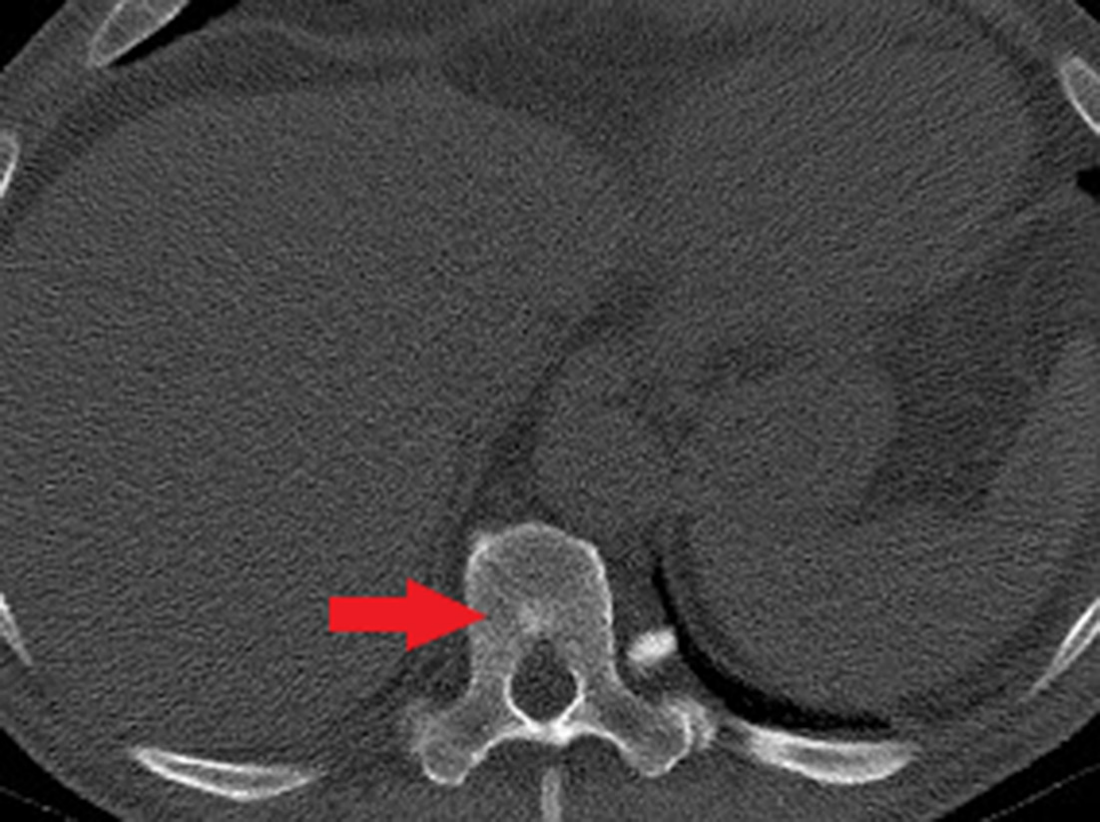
Axial CT image in case 2. Ill-defined sclerotic lesion with the T10 vertebral body (red arrow) incidentally detected on abdominal CT for an unrelated indication. Further investigation with bone scintigraphy was arranged.
